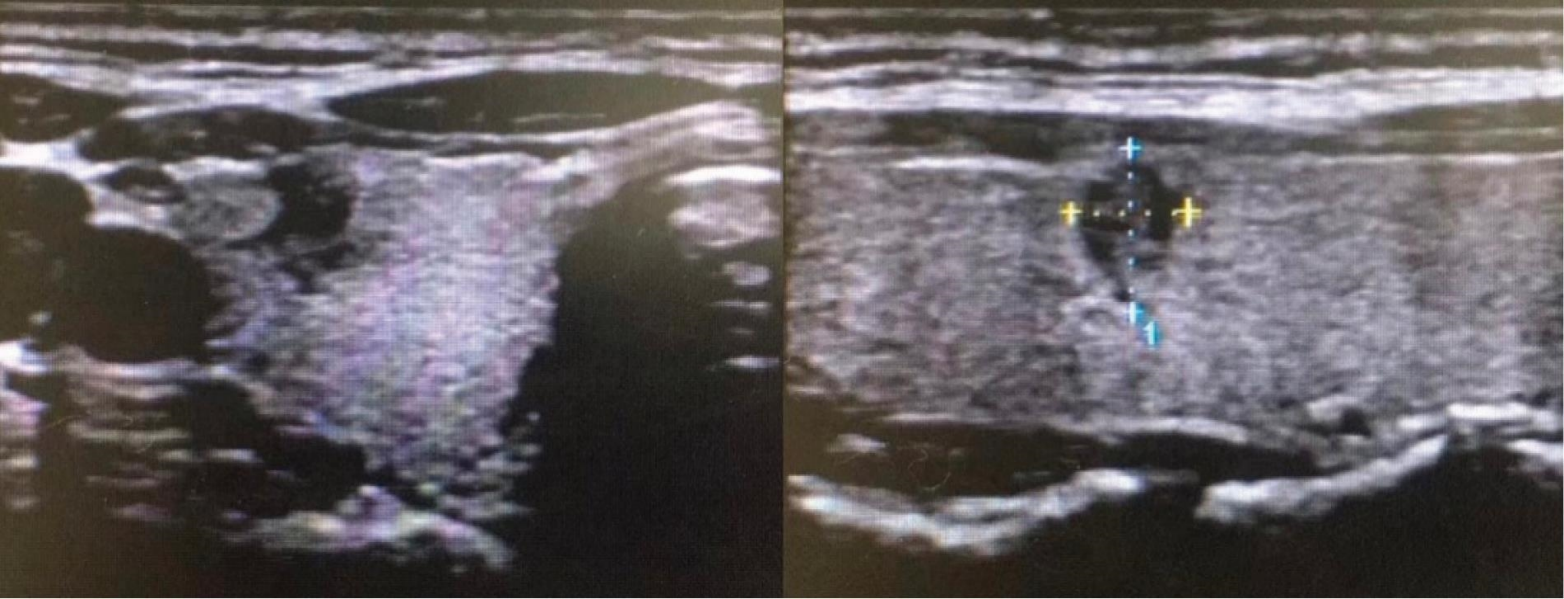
Ultrasound images of thyroid nodules.
